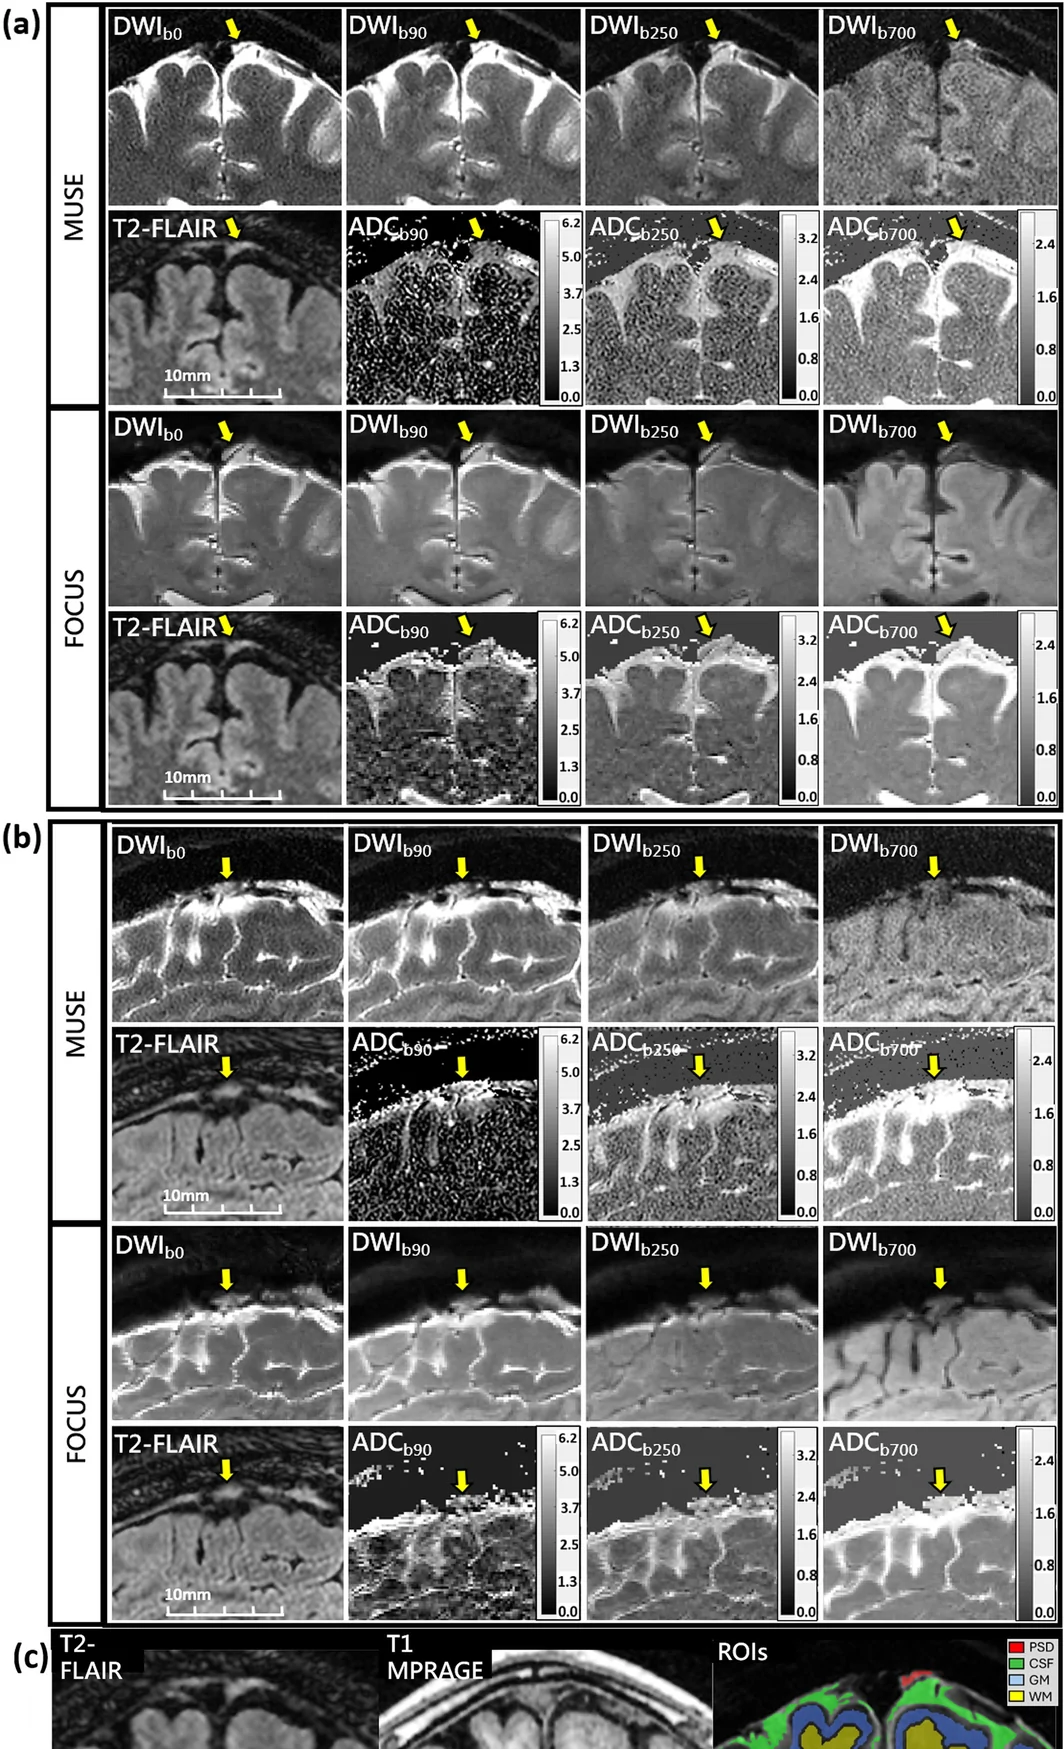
T2‐FLAIR, DWI (*b* = 0, 90, 250, and 700 s/mm^2^), and corresponding ADC maps acquired using MUSE and FOCUS methods in (a) coronal and (b) sagittal views. Spatial scale bars are shown in the FLAIR panels and also apply to the corresponding DWI and ADC panels within the same view. Intensity color bars are shown for the ADC maps, with ADC values expressed in ×10^−3^ mm^2^/s. The PSD (yellow arrows) becomes more clearly distinguishable from the adjacent CSF on higher *b*‐value ADC maps, particularly at *b* = 700 s/mm^2^, compared with lower *b* values ADC maps (*b* = 90 and 250 s/mm^2^). (c) T2‐FLAIR, T1‐weighted MPRAGE, and ROIs shown from left to right. ROI of PSD by manual draw and ROIs of CSF, GM, and WM using DWI coregistered to T1‐weighted images, the GM, WM, and CSF masks were eroded by two pixels to reduce the partial‐volume effects at tissue boundaries.
